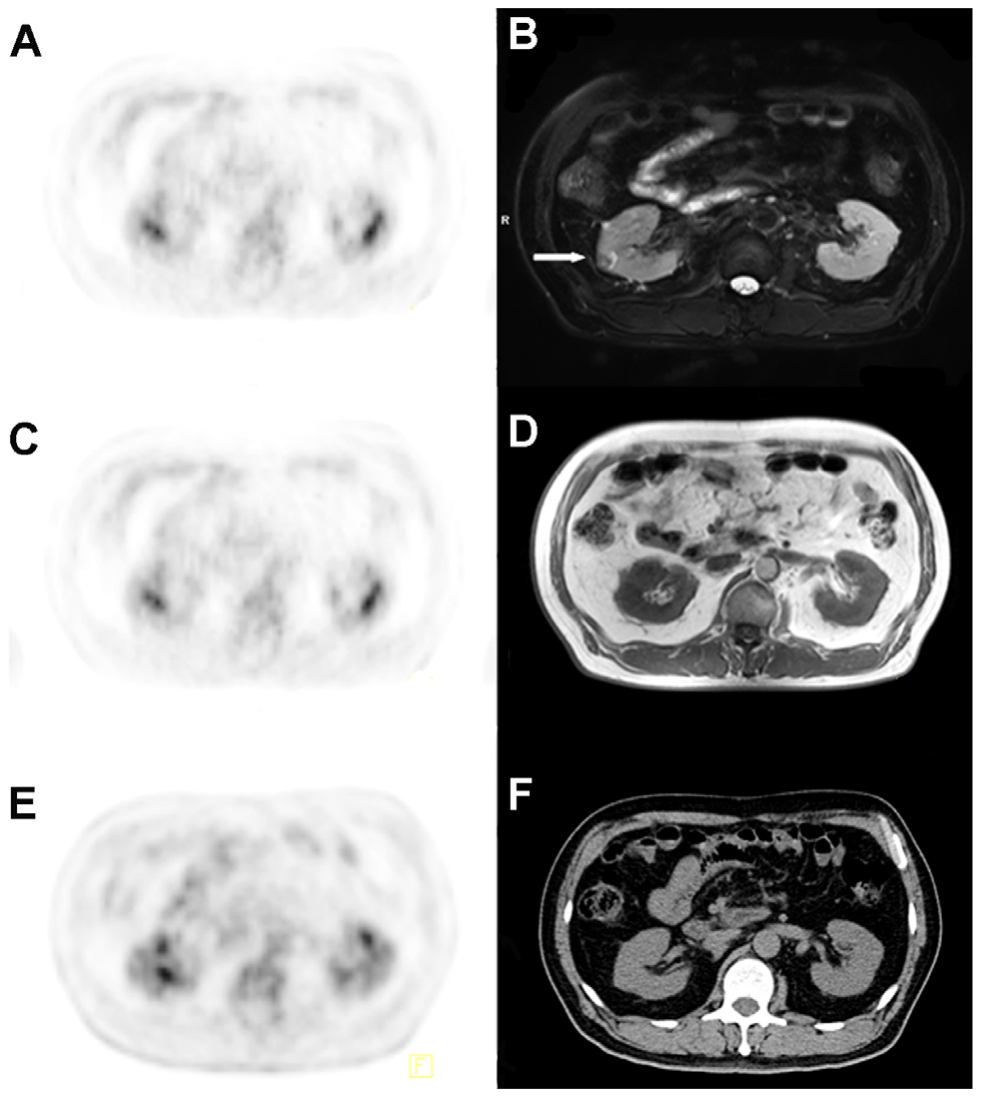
In a 52-year-old male, a right renal nodule was incidentally found by PET/MRI, and the renal lesion was later proven to be a renal cell carcinoma, Fuhrman II grade. T2WI-FS showed the lesion (B, *arrow*) much better than T1WI (D) and CT (F); PET (A, C, and E) was false negative.
